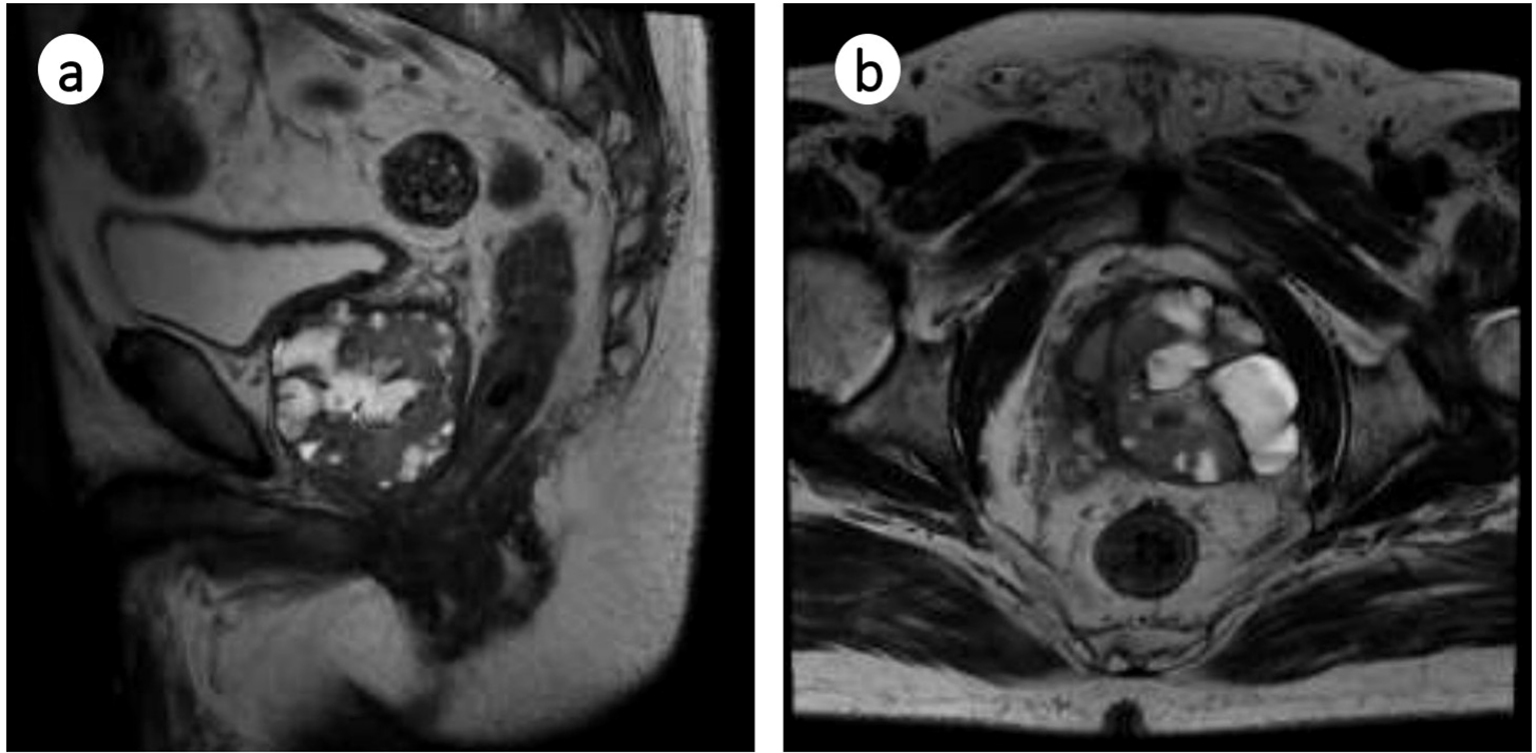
Contrast-enhanced MRI of the prostate. (a) Sagittal plane; (b) Transverse plane.
